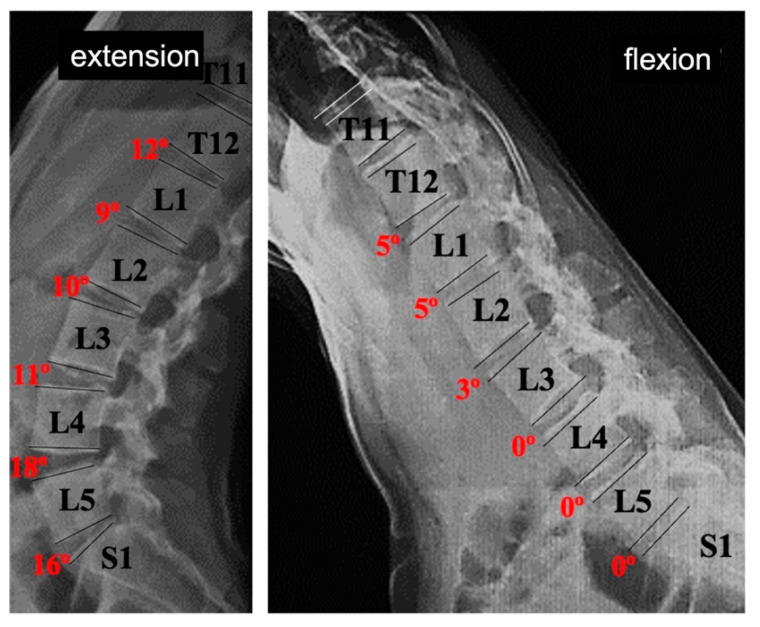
Fourteen-year-old girl with AIS type 1CN Lenke (54° Cobb) who underwent correction surgery with T3–L3 instrumentation. The images show the sagittal X-rays of the lumbar spine in maximal extension and flexion after removal of the implants.
